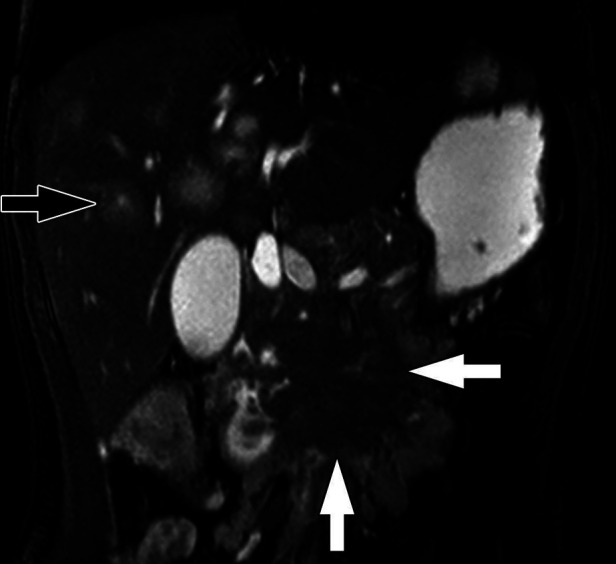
Pancreatic ductal adenocarcinoma. Coronal T2-weighted fat-saturated MRI of the abdomen shows a hypointense infiltrative mass involving the head and uncinate process of the pancreas (white arrows). There are additionally ill-defined hyperintense metastases in the liver (one indicated by the black arrow).
